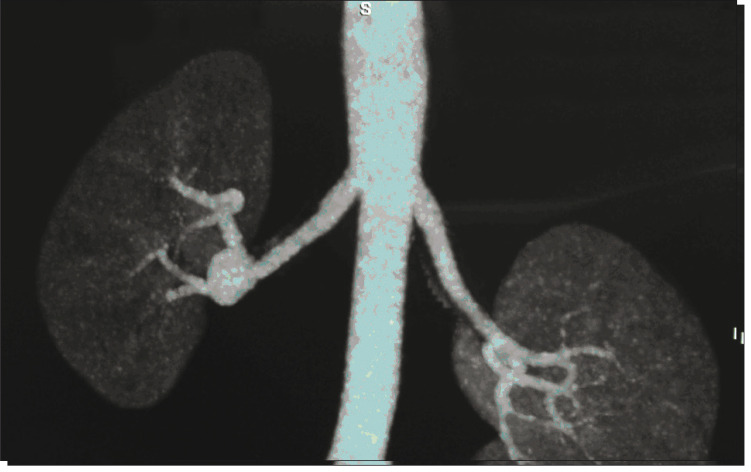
Computed tomography arteriography showing aneurysm of approximately 2.5 cm in the right renal artery.
